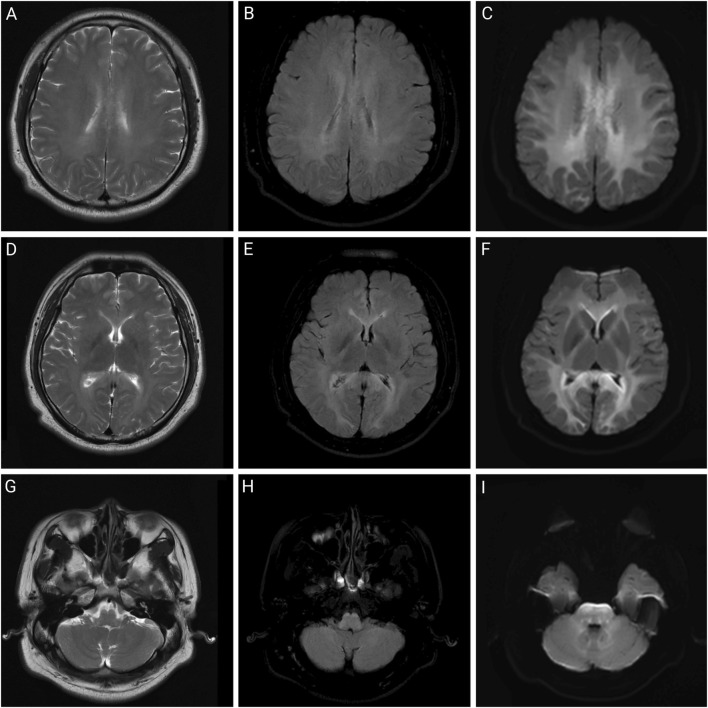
Brain MRI of patient two on day 2 of admission. Brain magnetic resonance imaging of a 32-year-old man after working in a workshop for 3 months. Abnormalities include symmetrical abnormal signals in the white matter areas of the bilateral cerebral hemispheres, corpus callosum, internal and external capsule areas, brainstem, and dentate nucleus. (A,D,G) are T2-weighted images; (B,E,H) are T2 fluid-attenuated inversion recovery (FLAIR) images; (C,F,I) are diffusion-weighted images.
